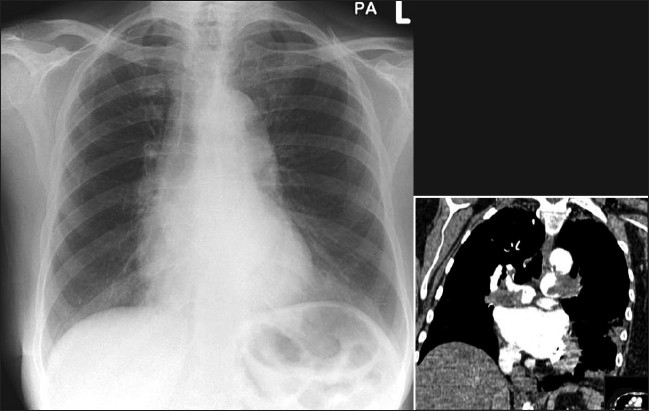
A frontal chest radiograph of a patient presenting with shortness of breath and hypoxemia, which shows no significant abnormality. However, CTPA (coronal reconstruction) shows extensive pulmonary embolism
